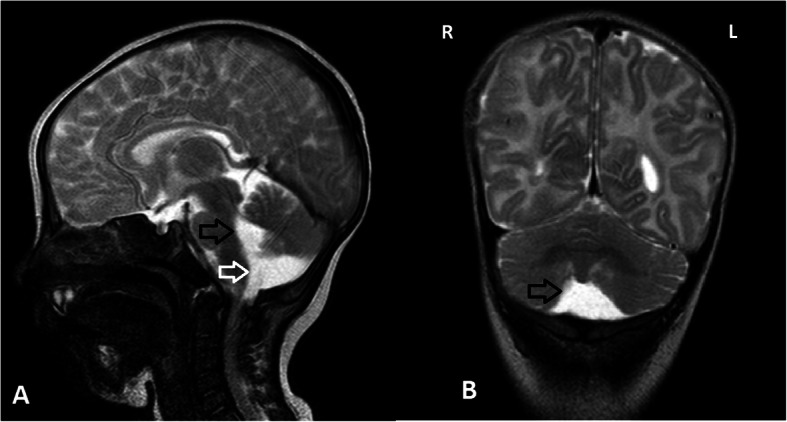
T2 weighted FSE Brain Magnetic Resonance Imaging. a. Sagittal scan: enlarged fourth ventricle (black arrow), and *cisterna magna* with inferior vermian hypoplasia (white arrow). b. Coronal scan: Enlarged *vallecula cerebelli* and hypoplasia of the cerebellar hemispheres (black arrow)
